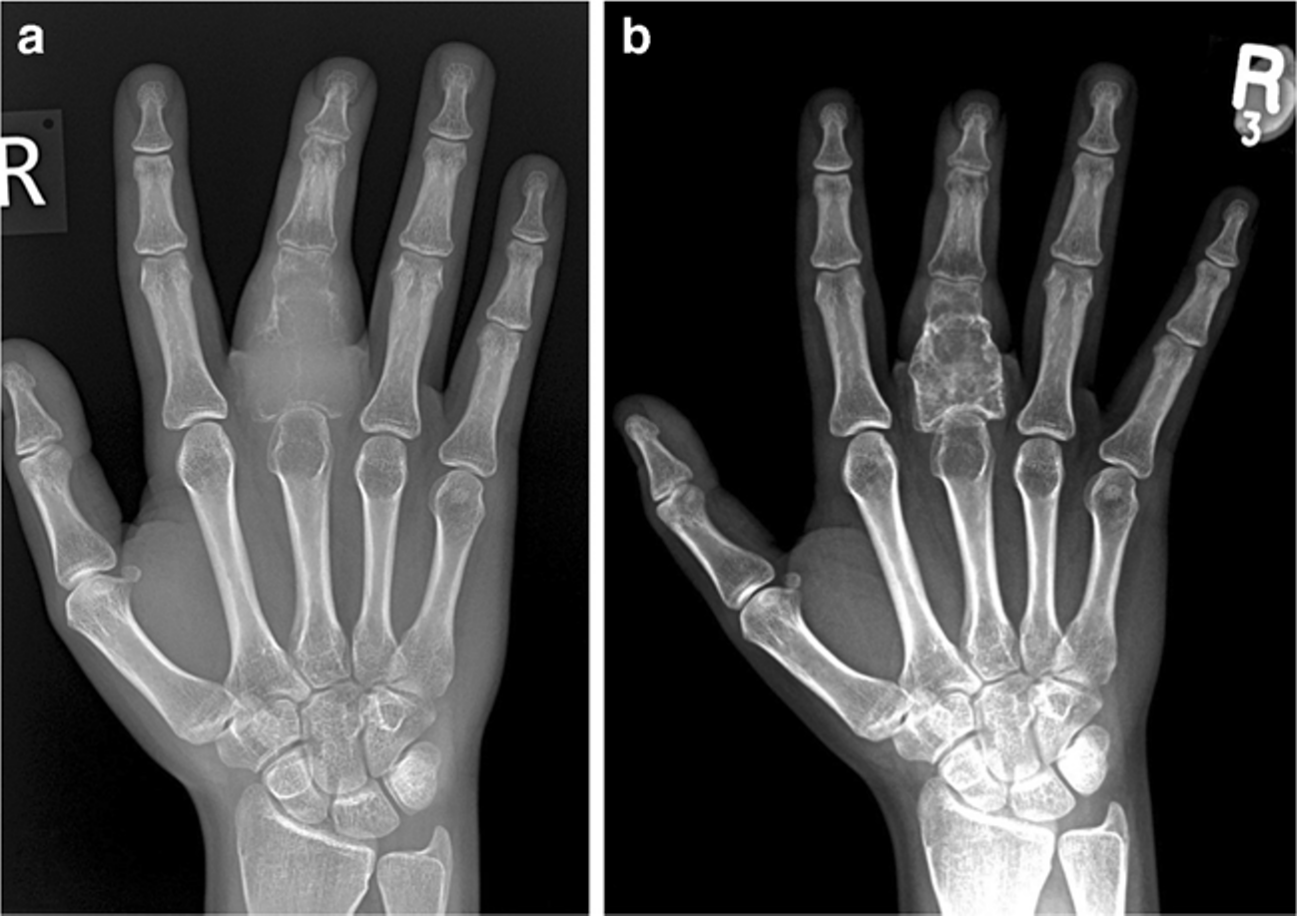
A 17-year-old girl presented with swelling of the middle finger. Plain radiographs at presentation (a) and after 12 weeks on denosumab (b). a At initial presentation, an expansile lytic GCTB is present in the middle finger proximal phalanx with cortical destruction. b After 12 weeks on treatment, the lesion has decreased in size with marginal neocortex formation and matrix sclerosis
